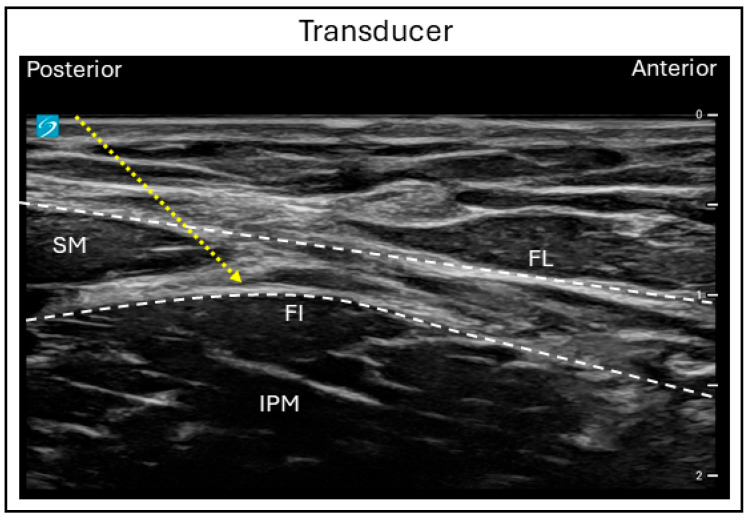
Ultrasound image demonstrating the in-plane infrainguinal fascia iliaca block technique. FL = Fascia lata; FI = Fascia iliaca; SM = Sartorius muscle; IPM = Iliopsoas muscle; Dashed line = Needle trajectory.
